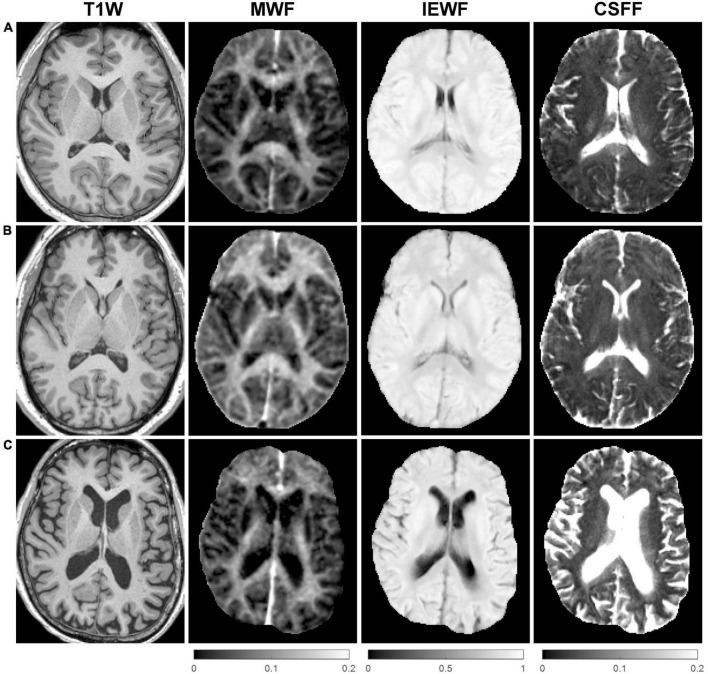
Representative axial mid-brain structural T1-weighted (T1W) magnetization-prepared rapid acquisition gradient echo (MPRAGE) images as well as corresponding myelin water fraction (MWF), intra/extra-cellular water fraction (IEWF), and cerebrospinal fluid fraction (CSFF) maps derived from fast acquisition with spiral trajectory and adiabatic T2prep (FAST-T2) data acquired from (A) a 22-year-old male, (B) a 46-year-old male, and (C) a 73-year-old female cognitively normal (CN) volunteers. Note the marked increase in cerebral atrophy, cortical loss, and lateral ventricular volume on the T1W image, and higher parenchymal CSFF values, in the latter subject compared to the younger subjects.
